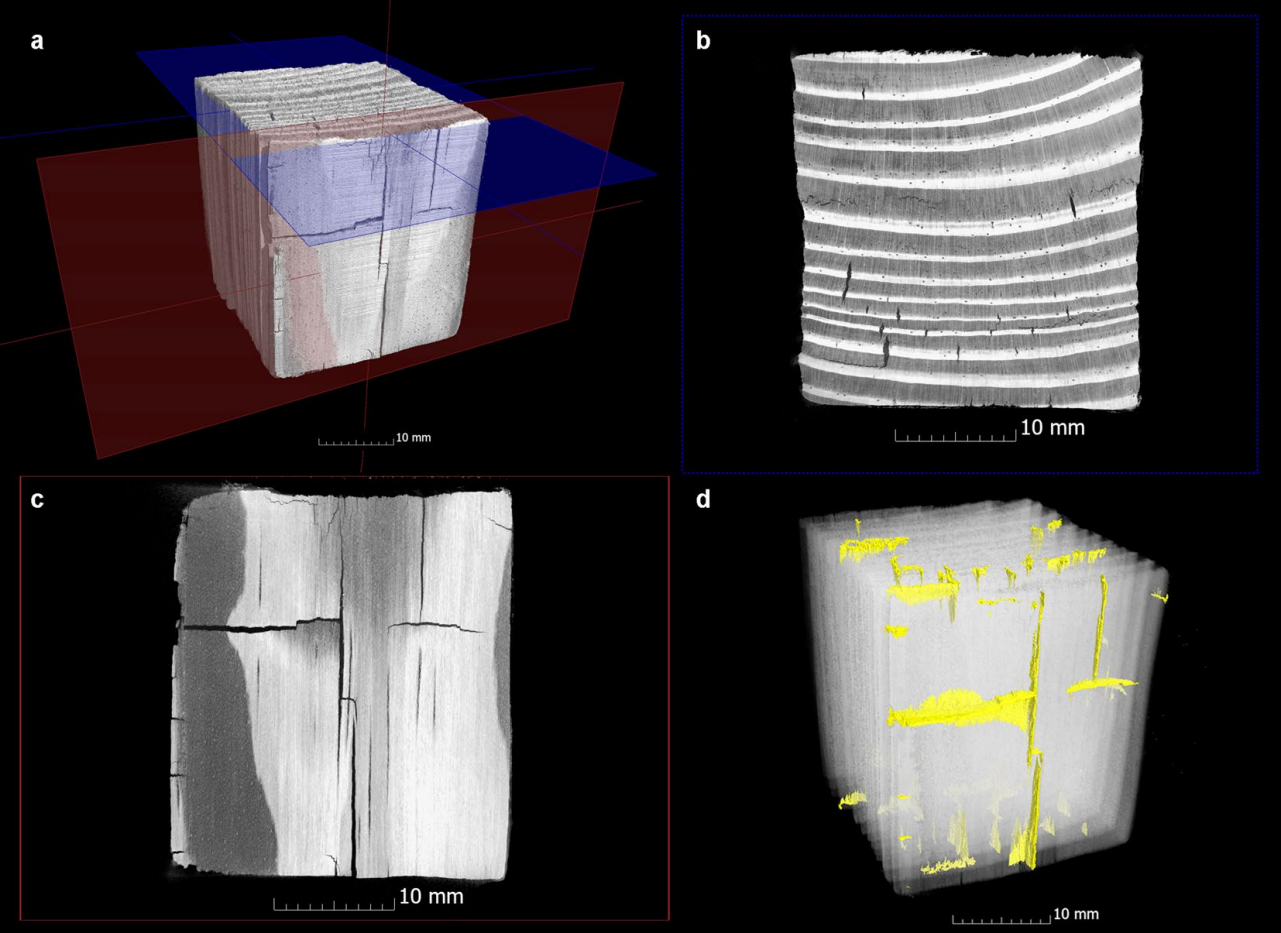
CT visualisation of the cracks in a pine sample (Pi-K800-2) conserved with Kauramin 800. The position of the cross section (b) and longitudinal section (c) are shown as blue and red planes in the 3D visualization (a). The 3D visualization (d) shows the cracks in yellow colour, which in this case only occur in the outer areas of the sample. In the cross-section (b), radial and tangential cracks can be seen, whereby collapse is also visible here in isolated cases. In the case of Kauramin 800, there is a transitional area between cracks and collapse and a clear separation is not always possible. In the longitudinal section (c), transverse cracks can be seen alongside radial cracks.
